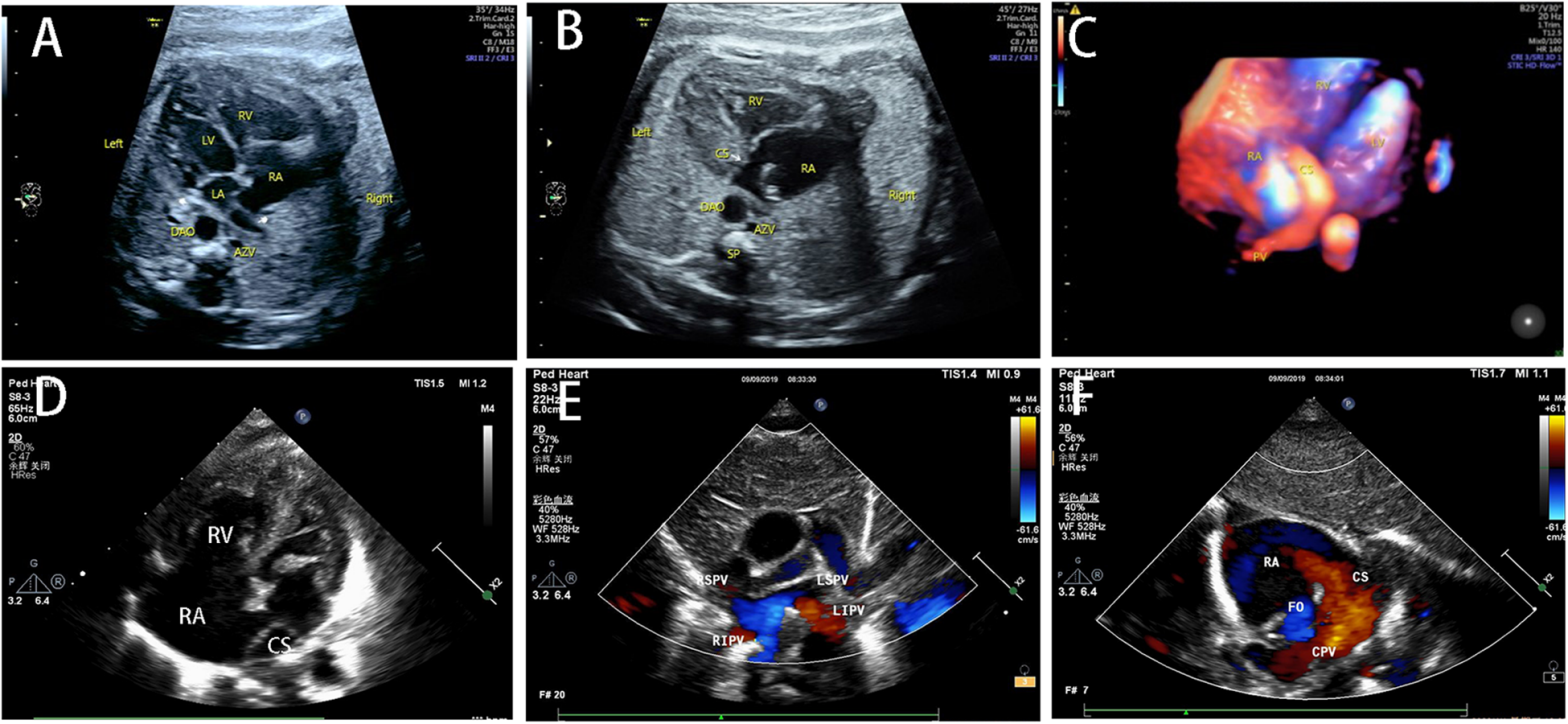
The prenatal and postnatal sonographic features of intracardiac TAPVC. (A) The four-chamber view showed ventricular discrepancy and no connection between PVs and LA. (B) The PVs drained into RA via dilated CS. (C) The entire drainage route of anomalous PVs. (D) The obvious asymmetry on the four-chamber view after birth. (E) All the PVs were not connected with LA. (F) The CPV drained into RA via dilated CS. TAPVC, total anomalous pulmonary venous connection; PV, pulmonary vein; LA, left atrium; RA, right atrium; CPV, common pulmonary vein; CS, coronary sinus.
